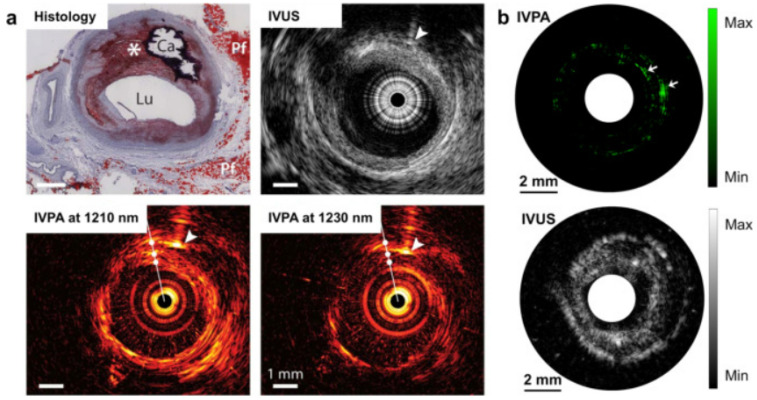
PA image of human artery in which dotted circle denotes the lipid-rich plaque [89]. (a) Intervascular photoacoustic (IVPA) and intervascular ultrasound (IVUA) image of an advanced atherosclerotic plaque in human (optical window 1.2 µm); (b) IVPA and IVUS imaging of excised human femoral artery (optical window 1.2 µm).
